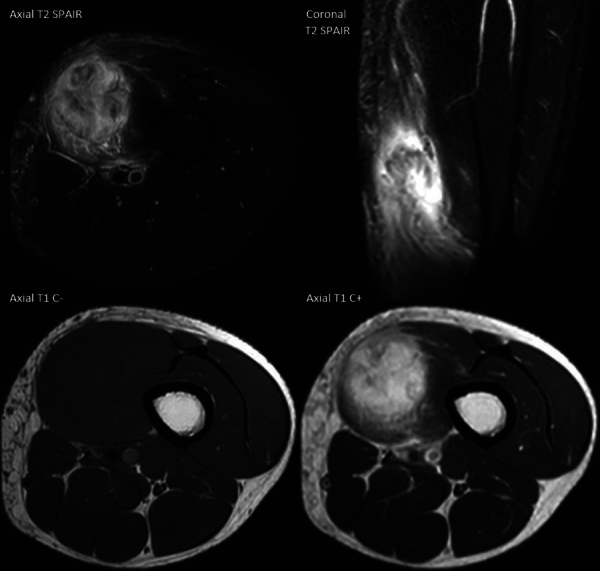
MRI of myositis ossificans shows marked muscle oedema and contrast enhancement.
